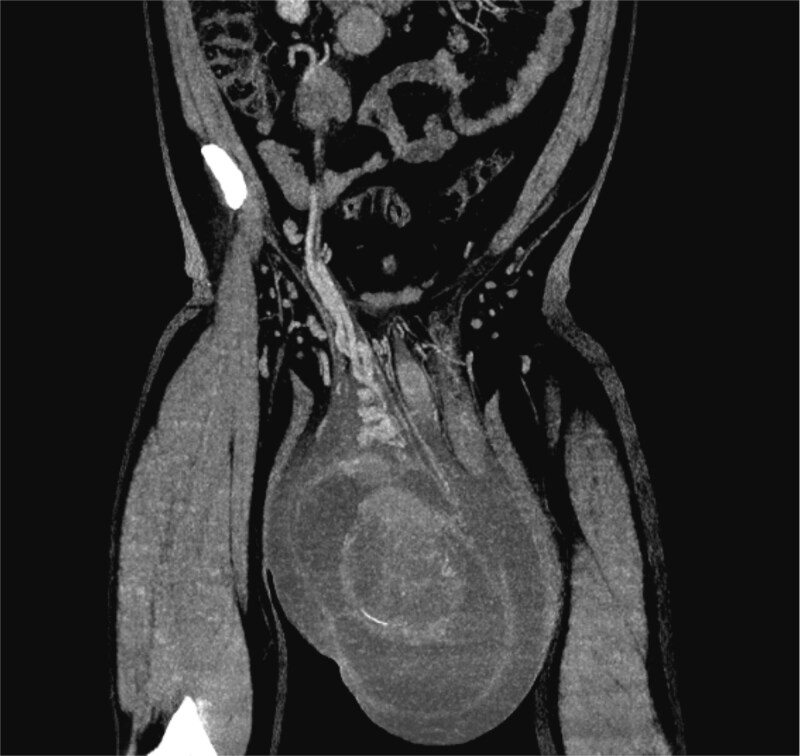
Contrast-enhanced coronal CT image demonstrating a large heterogeneous right testicular mass occupying the hemiscrotum, with associated hydrocele. The mass extends superiorly along the course of the right testicular vessels, with multiple enlarged retroperitoneal lymph nodes in the para-aortic and precaval regions, consistent witic spread.
